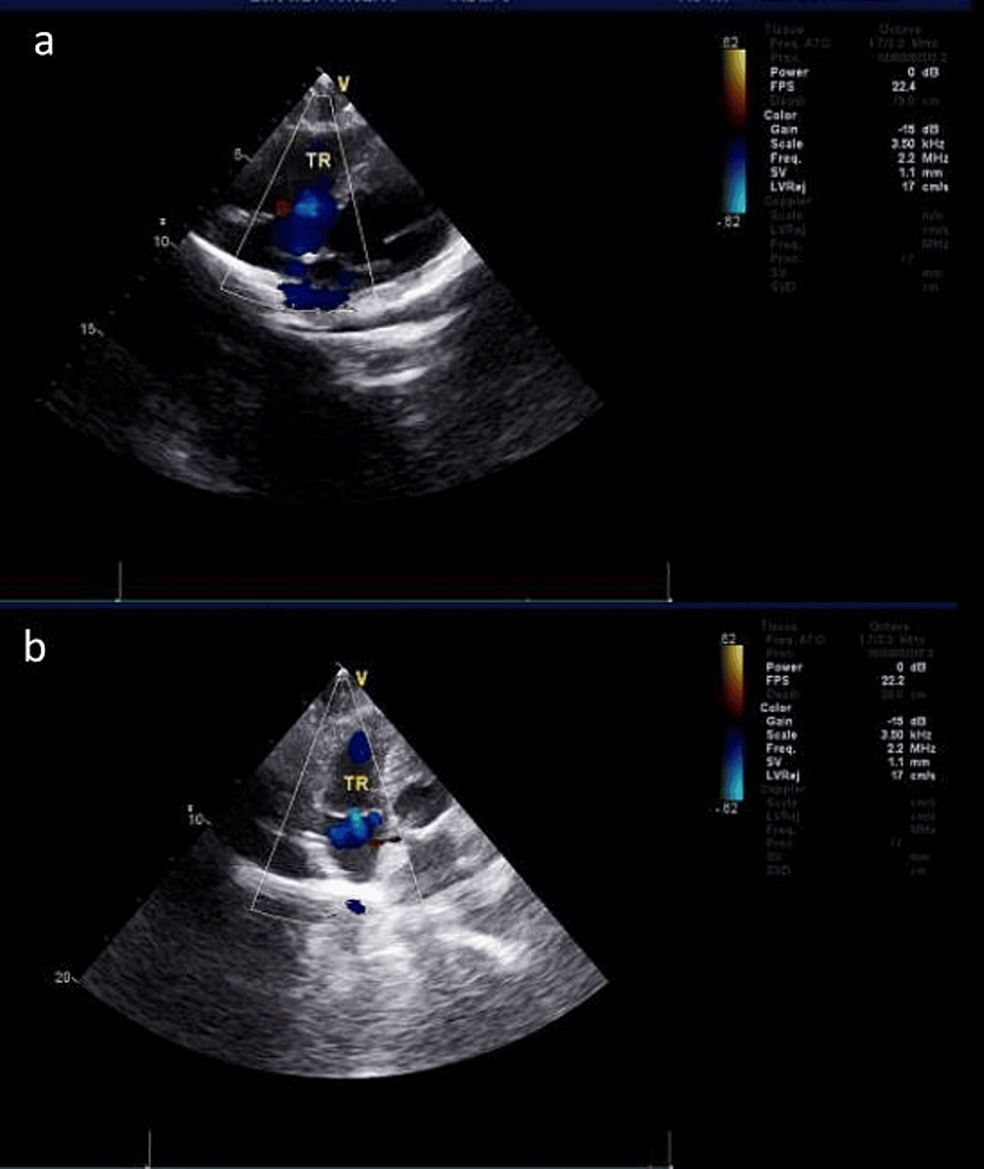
(a) Preoperative ultrasonic cardiogram (UCG): The heart size was within normal limits, with a left ventricular ejection fraction (LVEF) value of 66%. The tricuspid regurgitation velocity was 188 cm/s, the bundle length was 2.3 cm, the area was 1.8 cm2, and the volume was 1 ml. The pulmonary artery systolic pressure was 48 mmHg. (b) The UCG was followed up at six months, post-surgery. The heart size remained normal, with a slightly decreased LVEF value of 64%. No abnormalities were noted in the color of blood flow. The pulmonary artery systolic pressure decreased to 15 mmHg.
